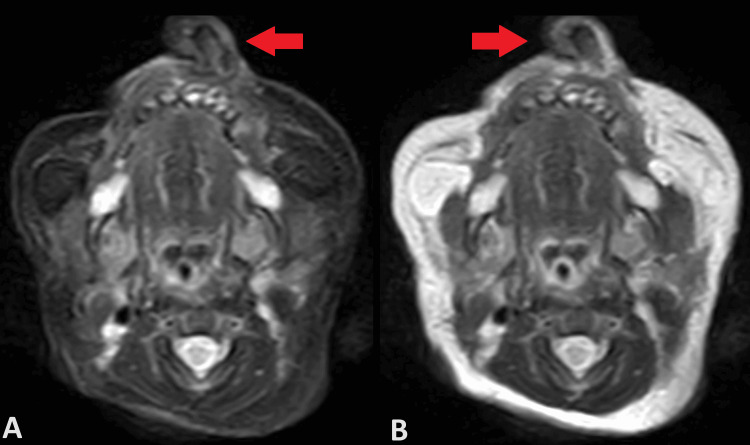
MRI brain plain (axial section) A) T2W fat suppressed scan image B) T2W image showing heterogenously enhancing soft tissue density lesion arising from maxilla with bony growth within. Features suggestive of teratoma.
